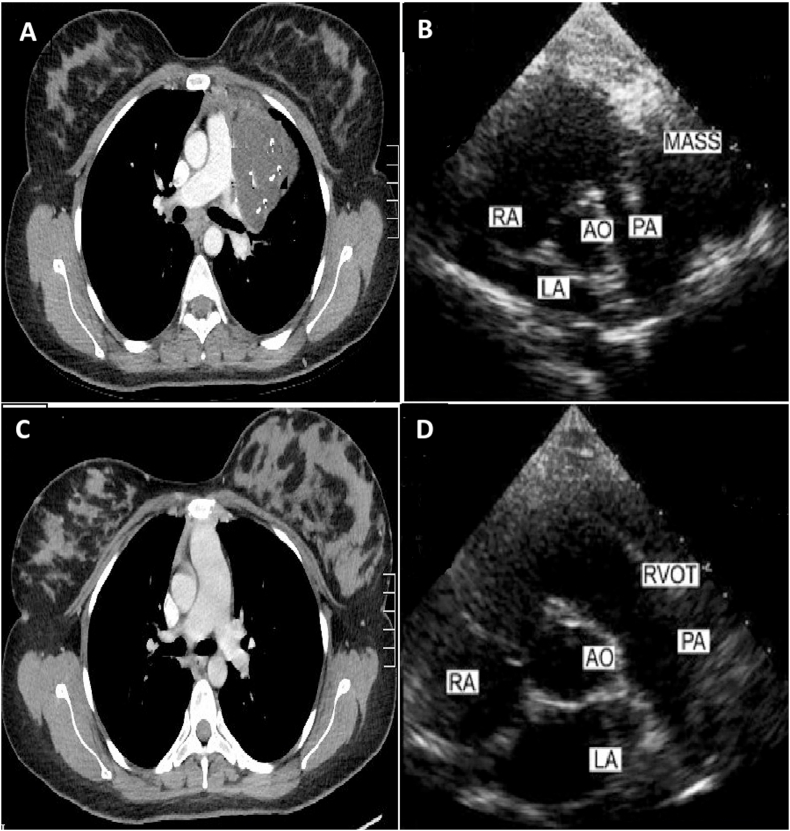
(A) CT scan of thorax showing anteruior mediastinal mass compressing the main pulmonary artery.(B) Preoperative ECHO demonstrate copreson of right venticular outflow tract.(C)Post operative CT can of thrax showing full expansion of pulmonary artery.(D)Post operative ECHO demonstrating release of right ventricular outflow tract obstruction.
